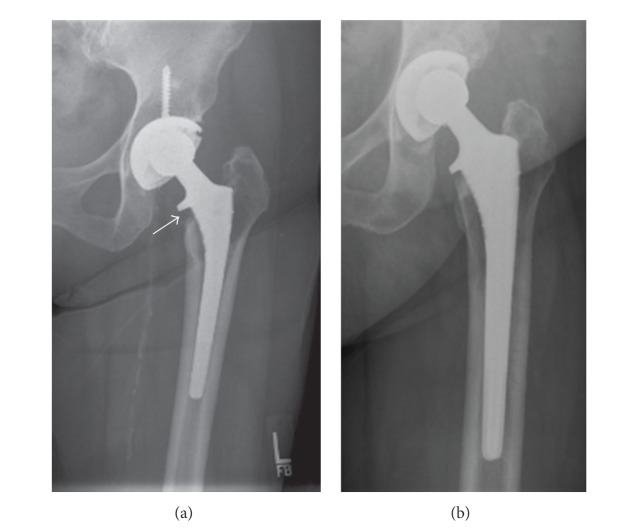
(a) AP radiograph of THA with machined polyethylene after 8 years of implantation. The measured polywear was of 4 mm. There is focal stem osteolysis and calcar erosion (arrow). (b) AP radiograph of THA with compression-molded polyethylene after 11 years of implantation. The measured polywear was of 2 mm. There were no significant signs of osteolysis.
